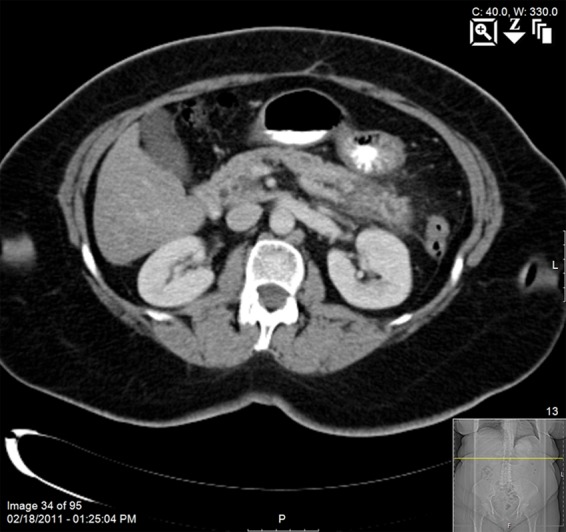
CT scan at initial presentation with acute pancreatitis in 2011.
